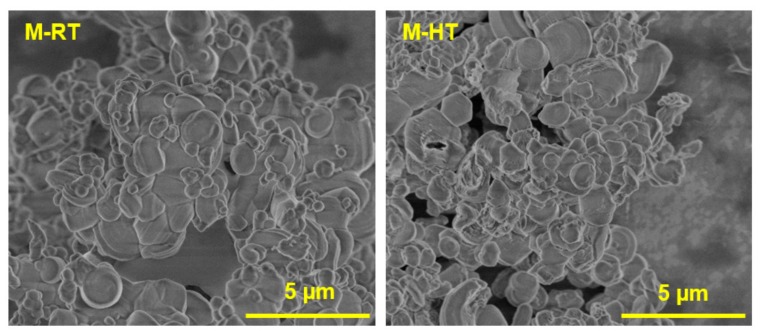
SEM images of the [CTA]-MCM-41 hybrid silicas.
